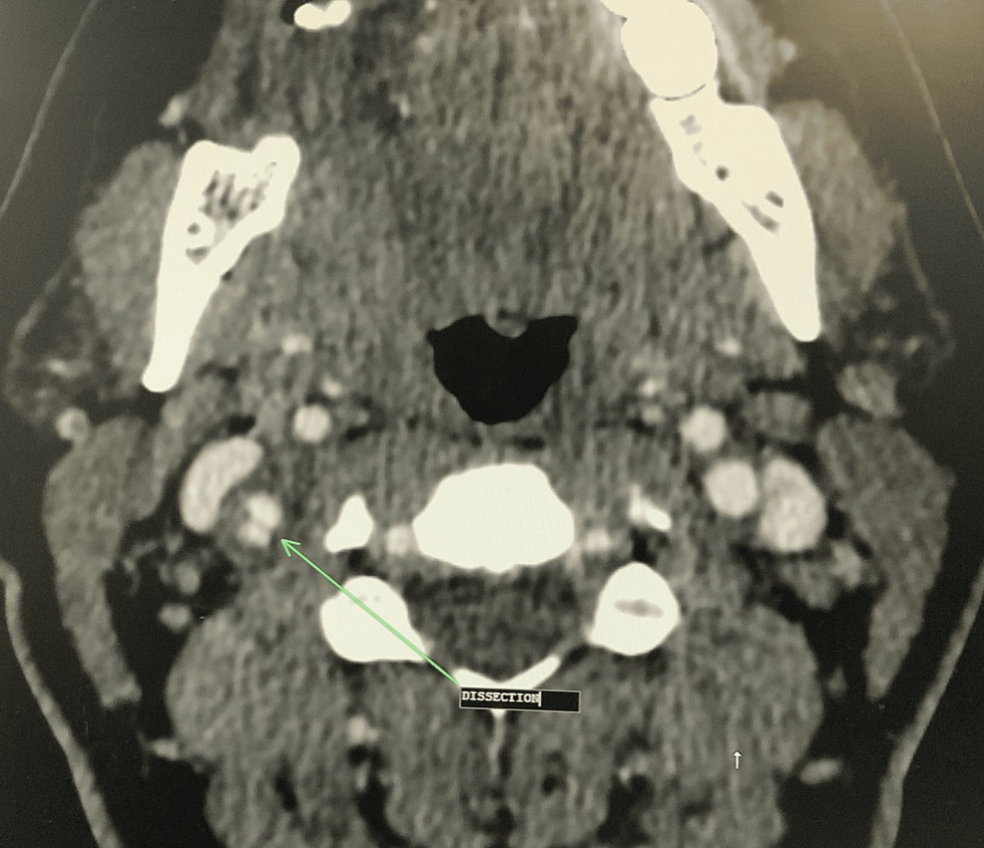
CT of the head showing right-sided carotid dissection (arrow) CT: computed tomography
